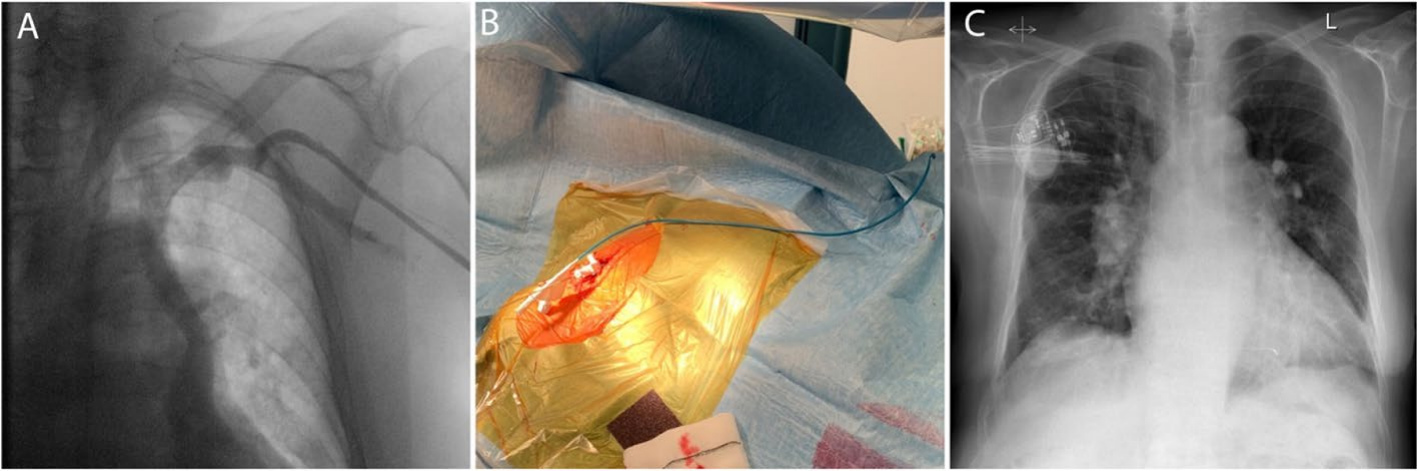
Images of chest x-ray fluoroscopy and manual modification of the C315-HIS delivery sheath. A A venography of left subclavian vein showing left superior persistent vena cava. B Reshaping point and the presumed septal region was estimated by placing the sheath on the body surface under fluoroscopy. C A chest X-ray of the final lead position
